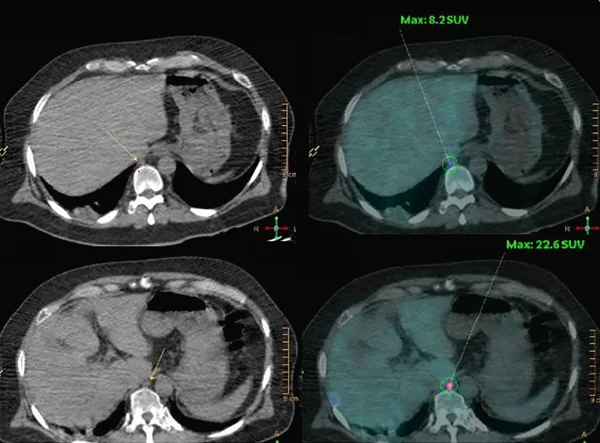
Preoperative PET‐CT demonstrated increased 18F‐FDG uptake in multiple pleuropulmonary and diaphragmatic lesions, including right subpleural nodules (SUVmax up to 33.9), a right upper lobe posterior lesion, a hypermetabolic right phrenic lymph node (SUV 22.6), and nodular thickening of the right diaphragm (SUVs 27.9 and 32.8), consistent with metabolically active metastatic disease.
